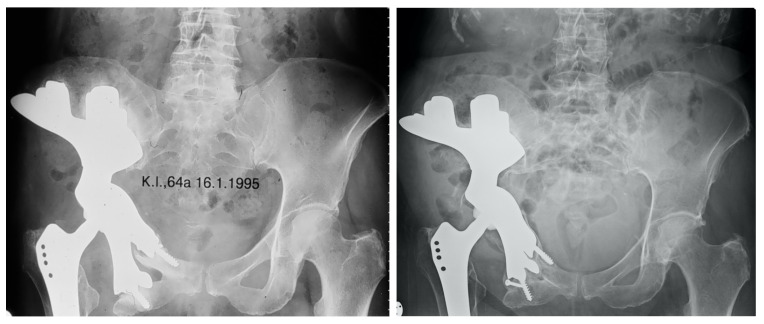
Patient 2 suffered from a periacetabular chondrosarcoma and received wide resection by a type II, III internal hemipelvectomy through a combined ventral and dorsal approach and subsequent reconstruction with a custom-made pelvic implant articulating with a Zweymueller stem in 1992. The patient suffered from aseptic loosening of the implant six years after primary surgery, which was addressed by accretion of iliac crest autograft bone. A deep vein thrombosis was treated conservatively. The X-ray, which was taken 20 years after primary surgery, shows good hip function, and the patient can walk with a walking stick and has an HHS of 89 points.
